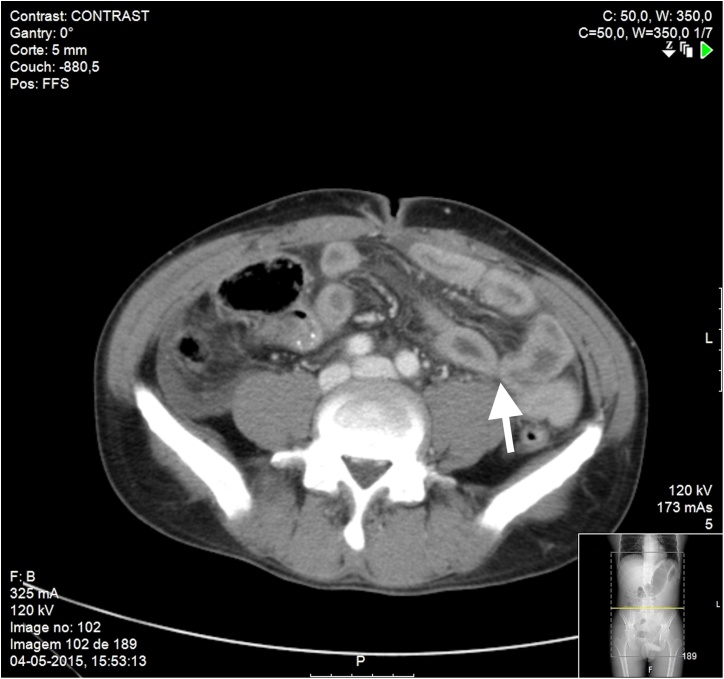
Abdominal CT: small bowel thickening (arrow).
